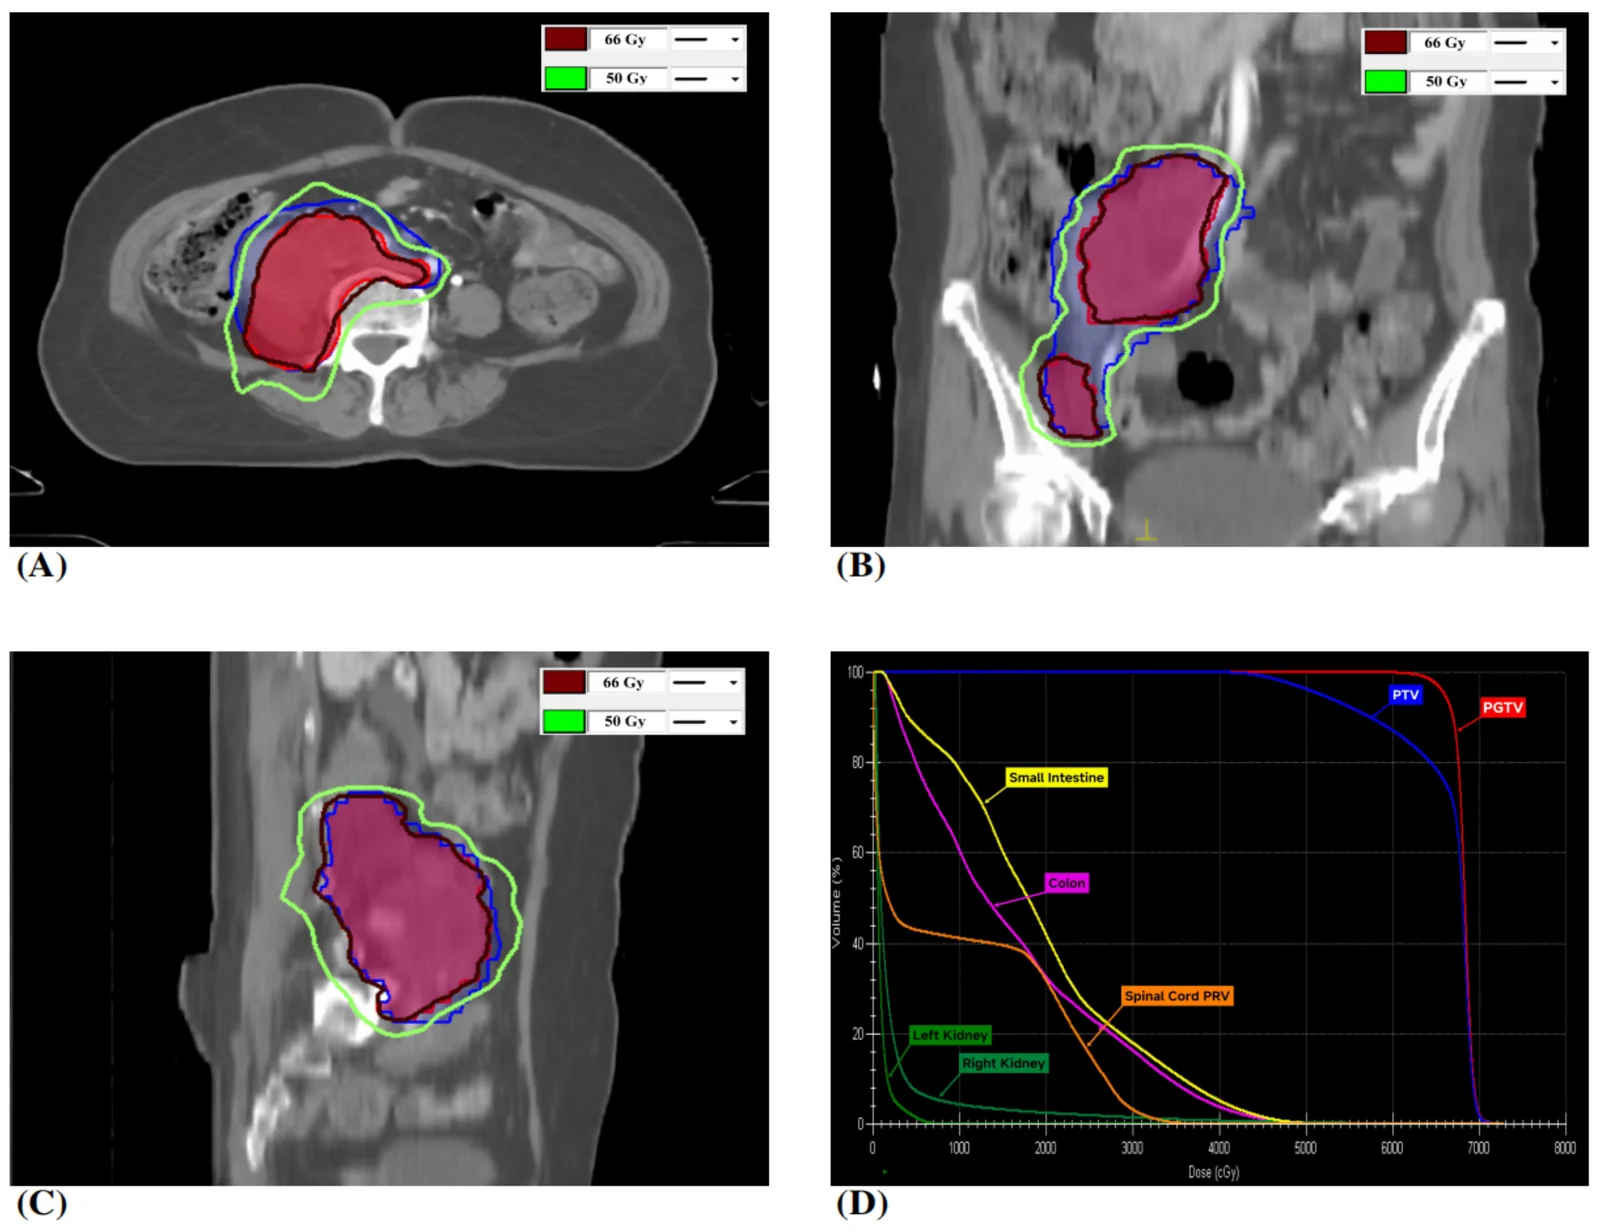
Radiotherapy plan for retroperitoneal IMT. (A) Axial CT slice showing PGTV (red) and PTV (blue). (B) Coronal CT slice showing PGTV (red) and PTV (blue). (C) Sagittal CT slice showing PGTV (red) and PTV (blue). (D) DVH showing dose distribution of target volumes and organs at risk.
